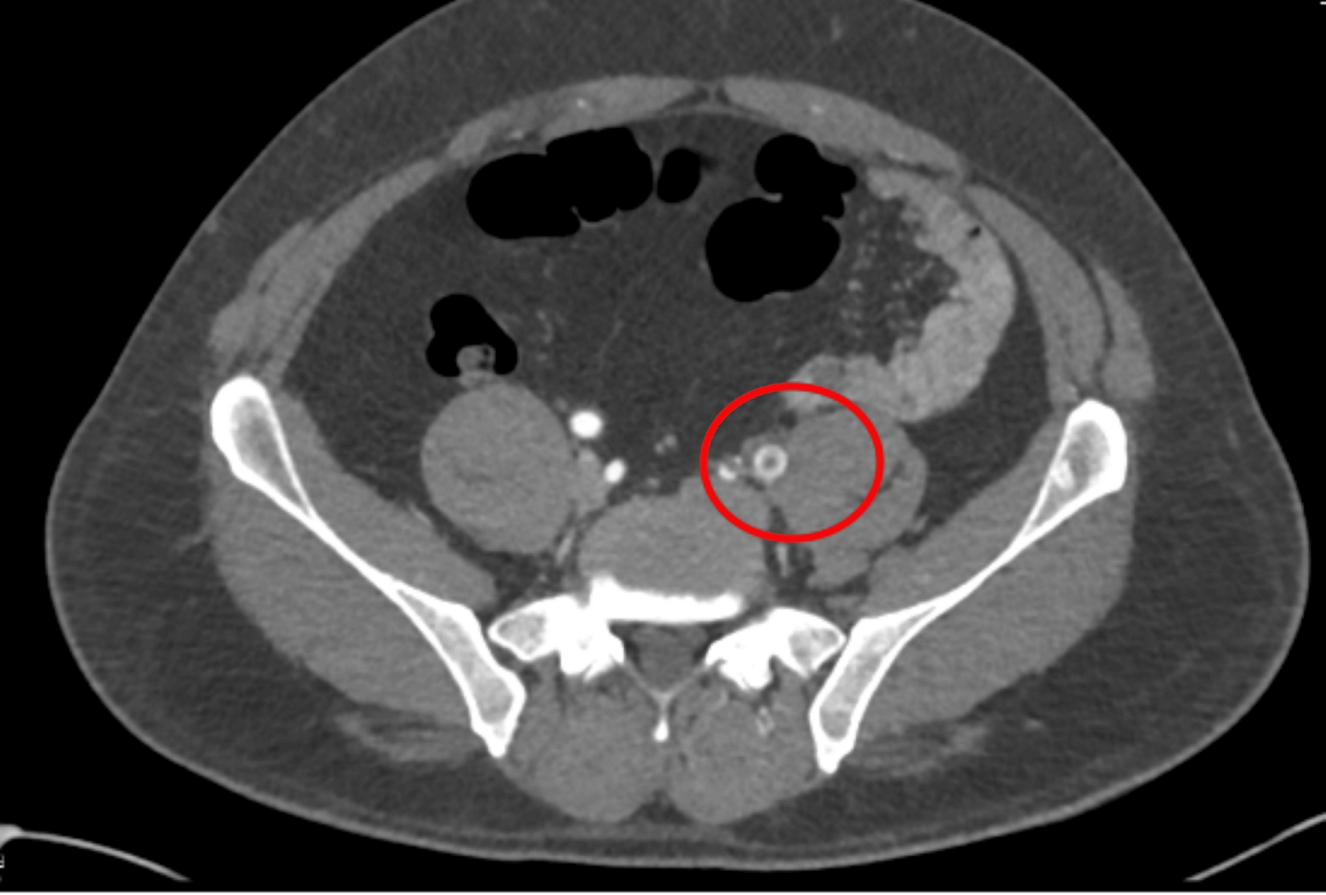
Initial CT angiogram showing a thrombus at the left iliac bifurcation CT: computed tomography
